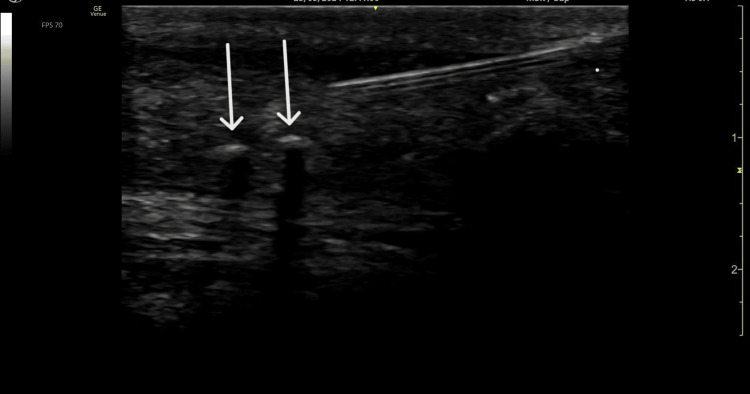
Ultrasound of the Achilles tendon of Patient 1 in long-axis view showing marked tendon thickening with anechoic voids and intra-tendinous calcifications consistent with tendinopathy and post-operative change.
